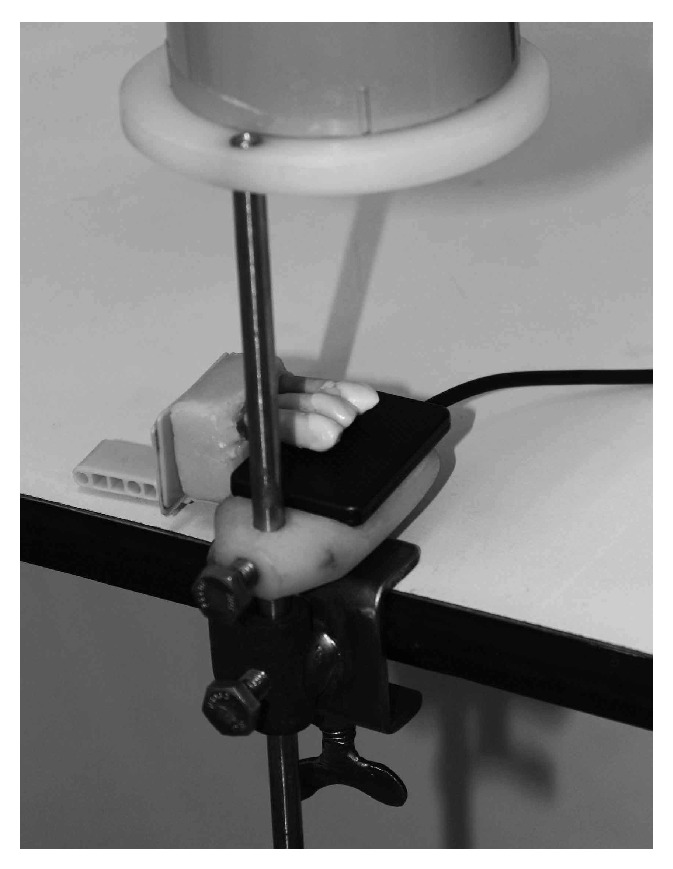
Device used to obtain interproximal X-rays.
